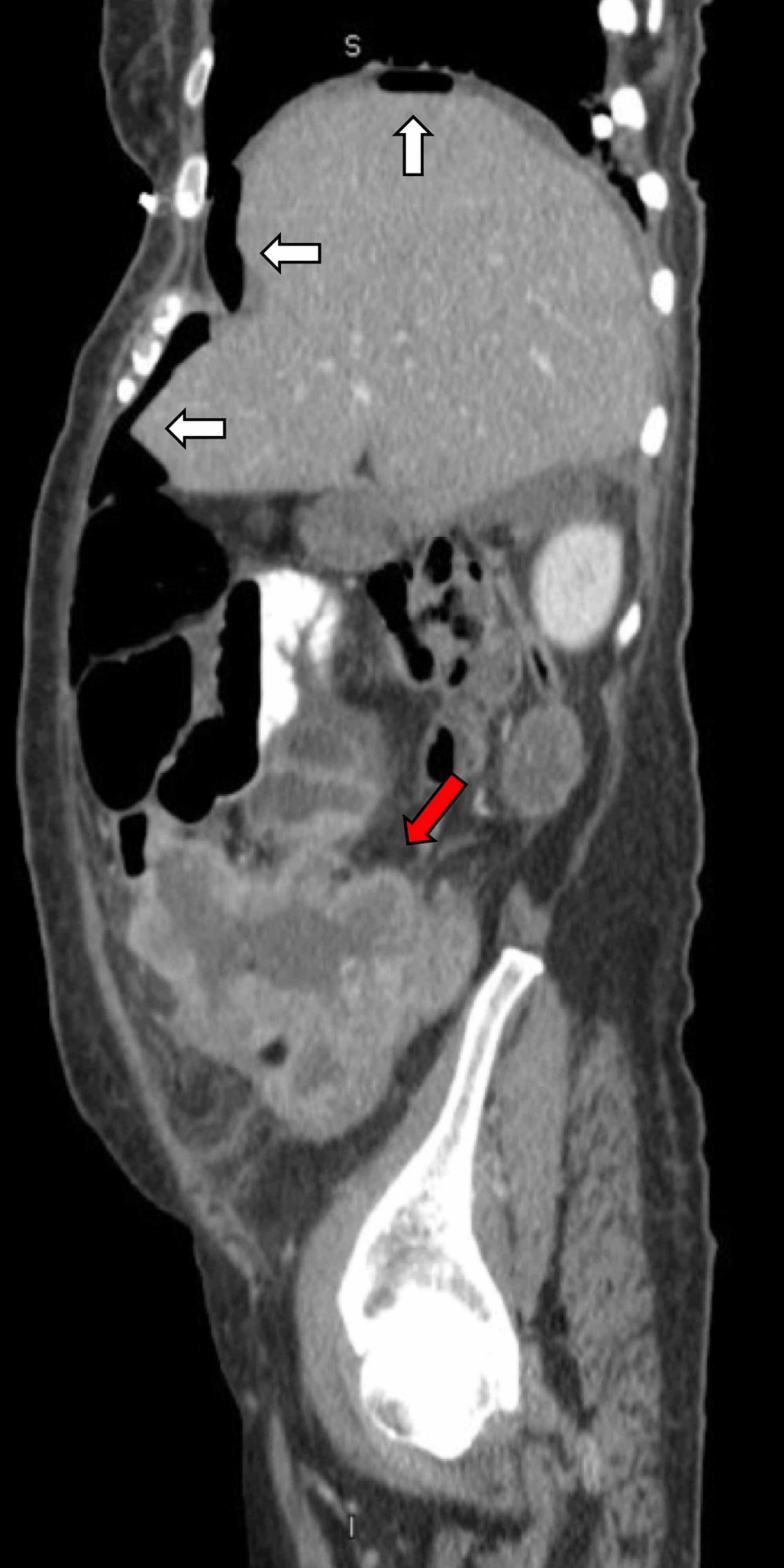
CT scan of the abdomen Pneumoperitoneum is shown by white arrows; irregular bowel thickening was noted in right lower quadrant (red arrow).
